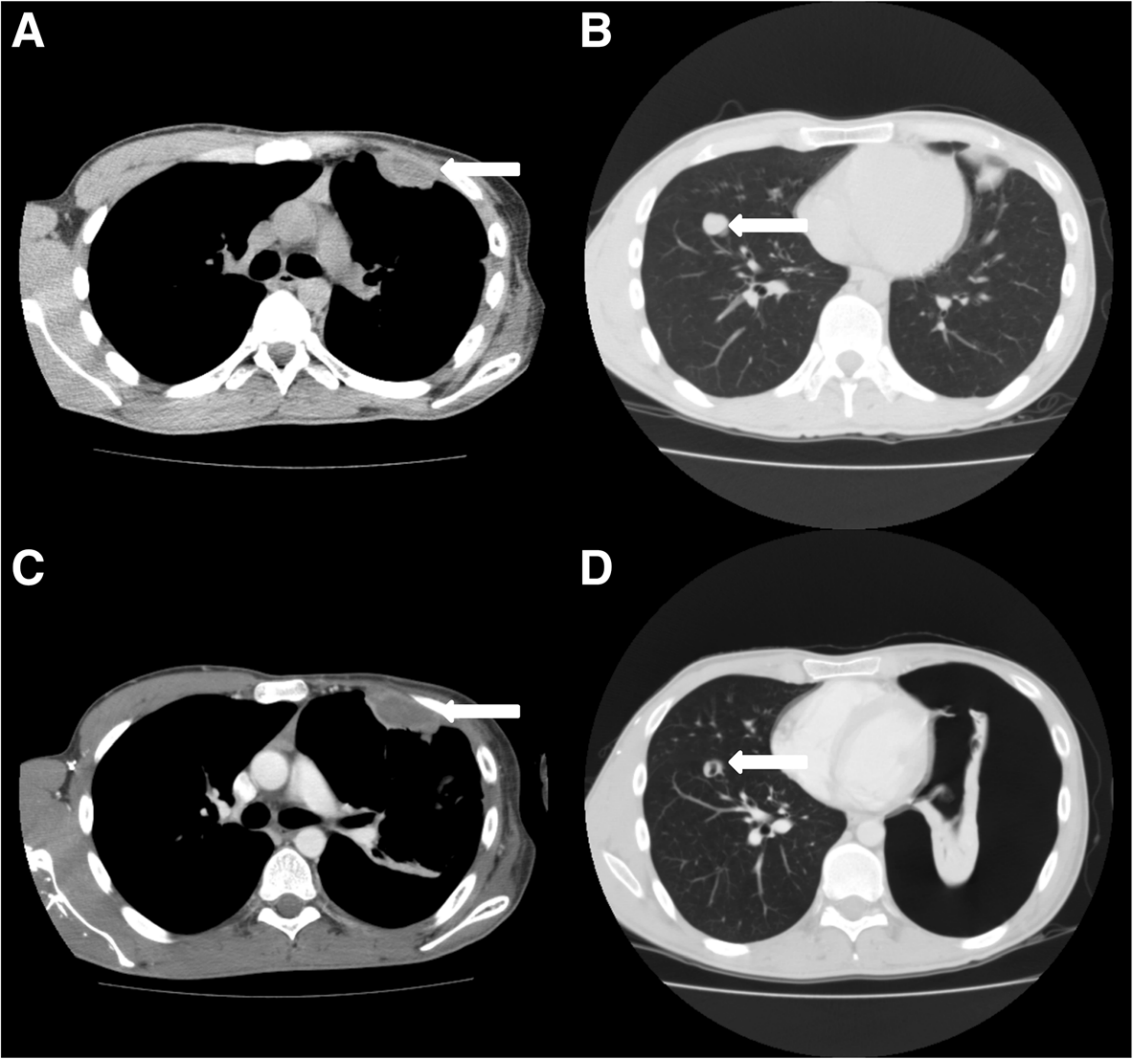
CT scans of patient 1. A and B: CT scan of the thorax at start of pazopanib, showing a pleural metastasis of the left lung with central hypodensities suggesting necrosis (A, arrow) and showing a pulmonary metastasis in the right lung (B, arrow). C and D: CT scan, when presenting with a left-sided pneumothorax (D), showing progressive hypodensity of the pleural metastasis on the left side (C, arrow) and central cavitation in the metastasis in the right lung (D, arrow).
